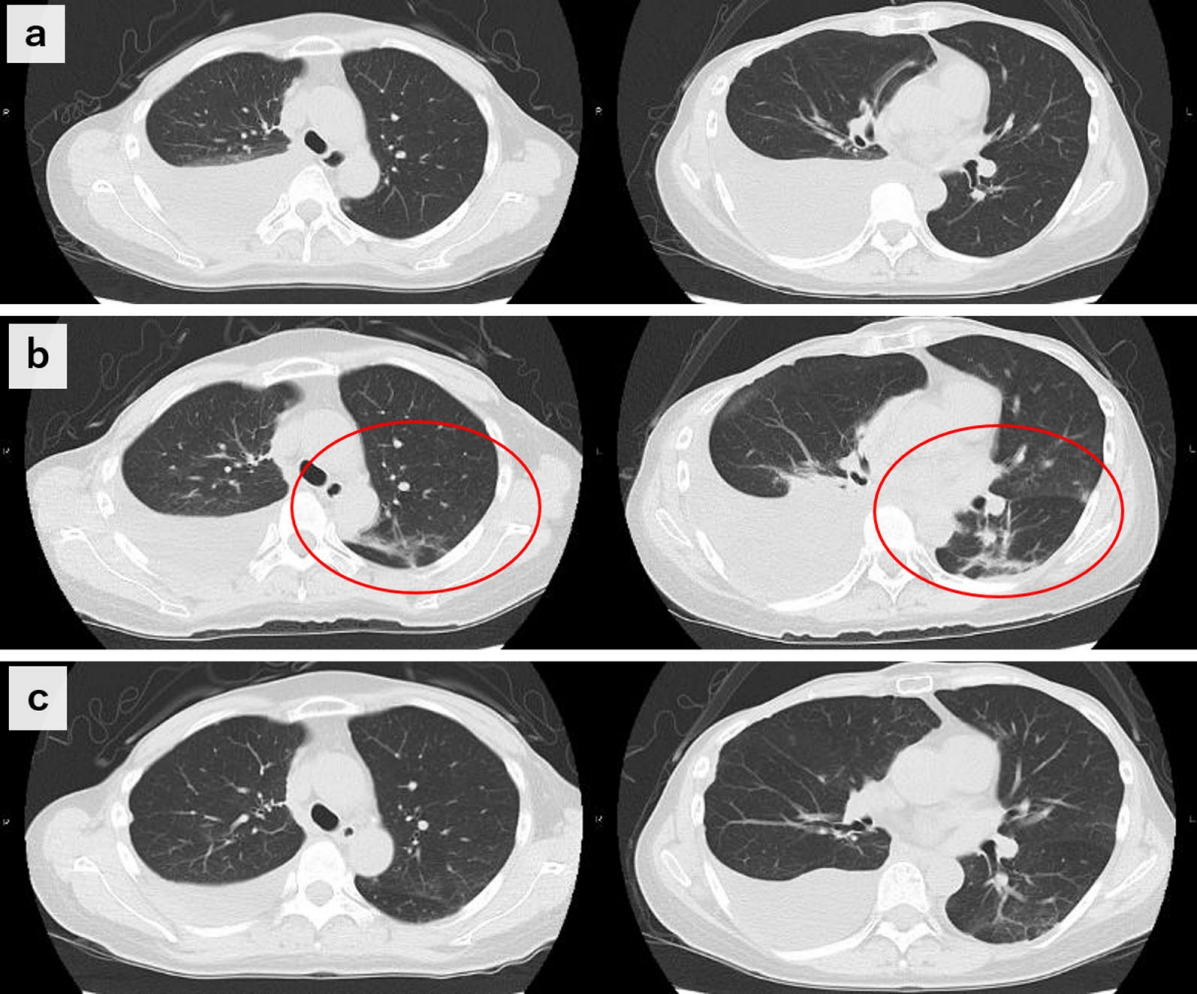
CT scan findings over the clinical course. a Day of hospitalization; b 7 days after hospitalization; c 2 weeks after hospital discharge. Red circles indicate pneumonia
